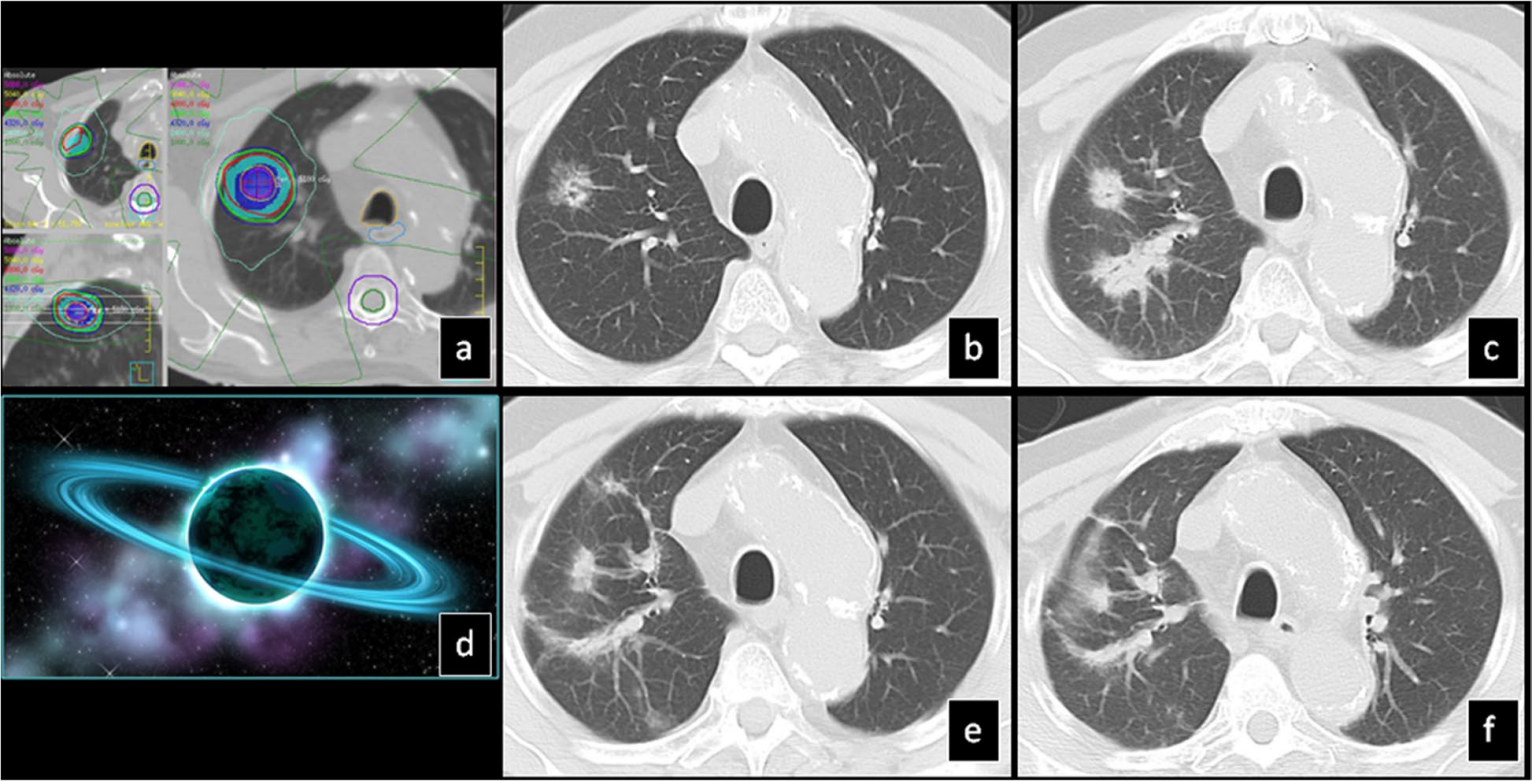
SBRT-treated lung cancer continues to decrease in size at 19 months: a) Isodose multiplanar images for planning. b) Pre-SBRT CT shows a part-solid nodule. c) 6 mo post-SBRT the size is stable, however the nodule is denser and there is surrounding focal peribronchial consolidation away from the nodule. d, e) 10 mo post-SBRT there is decrease in size of the primary lesion and development of homogeneously thick curvilinear density at a radius away from the center of the nodule in keeping with the “orbit-sign” (d). f) 19 mo post-SBRT there is further decrease in size of the primary lesion and mild shrinkage of the surrounding “orbit-sign”
